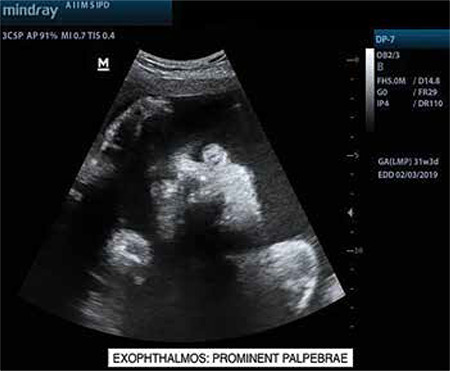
Ultrasound image (2-dimensional) of coronal view of fetal face demonstrating easily visible eyeballs and palpebrae, suggestive of orbital proptosis
